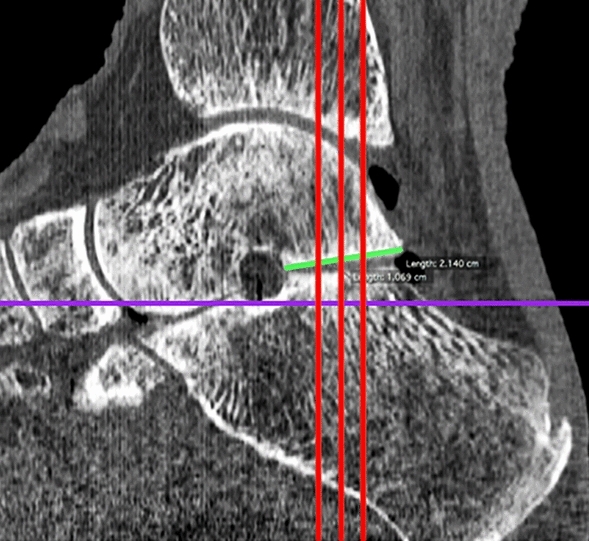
Sagittal CT view demonstrating the definition of the three coronal planes—anterior, middle, and posterior—used for measurement of subtalar vertical angle (SVA) and talar subluxation
